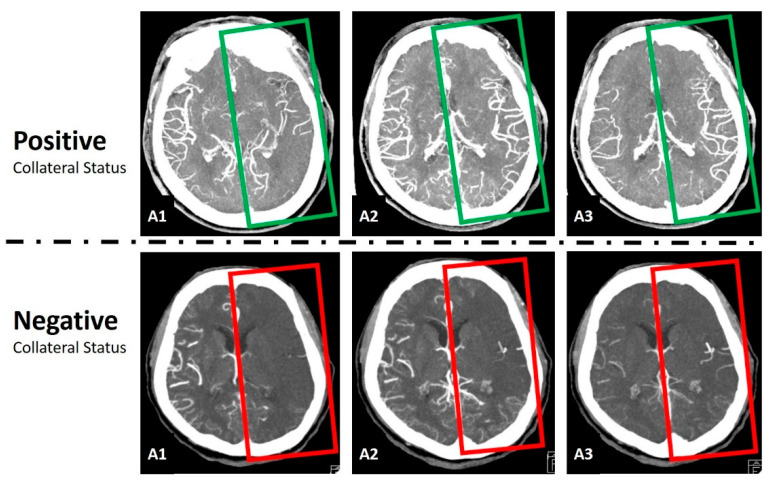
Demonstration of positive and negative collateral status on multiphase CTA. The A1, A2, and A3 indicate the three arterial phases. In the positive collateral status (upper row), the left side brain (green frame) is the ischemic side. When the enhancement of the arterial branches in the ischemic side can achieve similar appearance to the A1 contralateral normal side in A1 or A2 (this case), the collateral status is good or intermediate, respectively, and the result is defined as the positive collateral status. On the contrary, in the negative collateral status (lower row), the arterial branches in the ischemic side (red frame) cannot be enhanced like the A1 contralateral normal side in A1 or A2, suggesting that the collateral status is poor and the result is defined as the negative collateral status.
